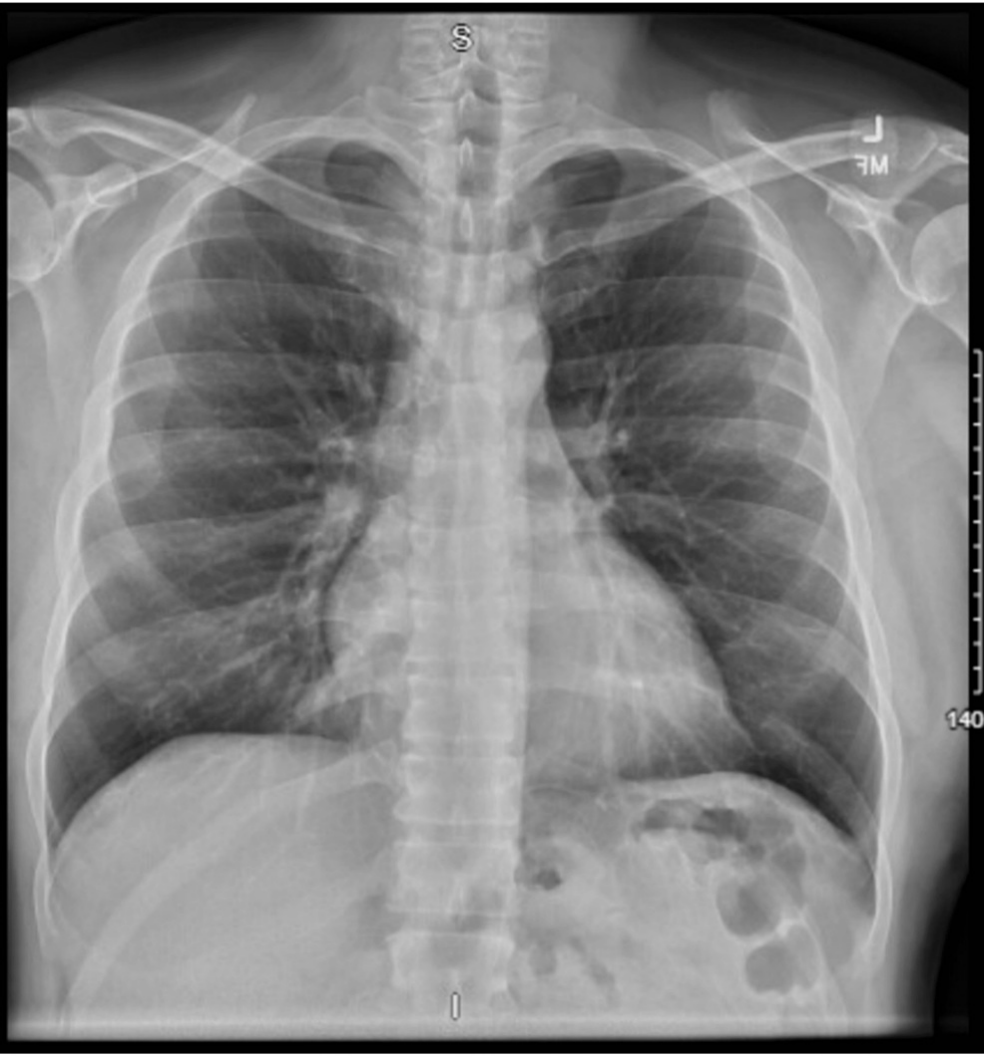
Chest X-ray - no acute pathology
